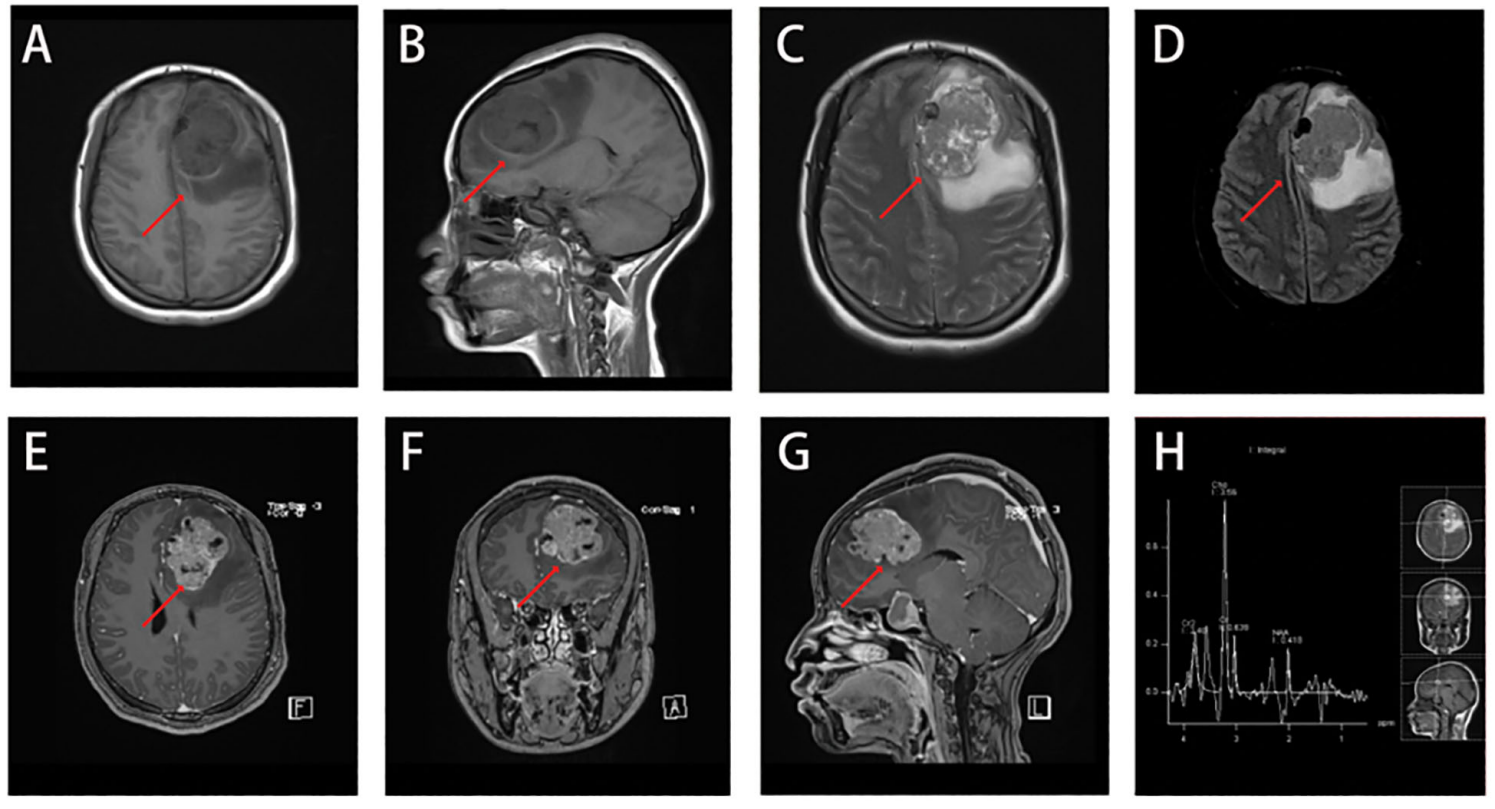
Irregularly shaped occupying lesion in the left frontal lobe, consider glioma or vascular ependymoma, metastatic tumor not excluded. (A-C) A large lobulated mass was seen in the left frontal lobe, measuring about 41×35×54 mm, with a slightly long T1 and long T2 signal, and the internal signal was not homogeneous. And there were multiple patches of long T1 and long T2 signal, nodular long T1 and short T2 signaling is seen in the medial margins. (D) Slightly high signal in FLAIR, the medial margin of FLAIR showed low signal, surrounded by edema. (E-G) The enhancement scan showed obvious heterogeneous enhancement, with more obvious enhancement of the medial margin of the nodule, and the midline structure was shifted to the right. (H) MRS suggested that the Cho peak in the area of interest was obviously elevated, and the NAA peak was reduced and partially disappeared.
